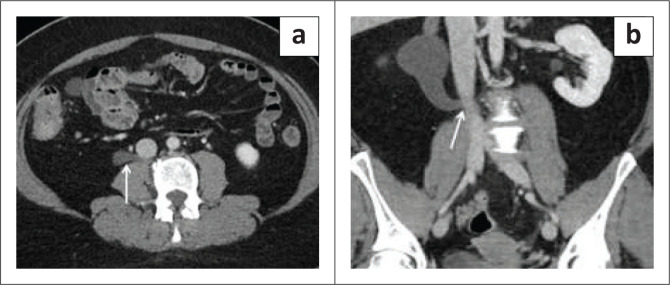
A 47-year-old female with urinary tract infection and haematuria demonstrated right-sided hydronephrosis on ultrasound. Axial post-contrast CT (a) and coronal (b) images demonstrate a retrocaval course of the ureter (arrow).
